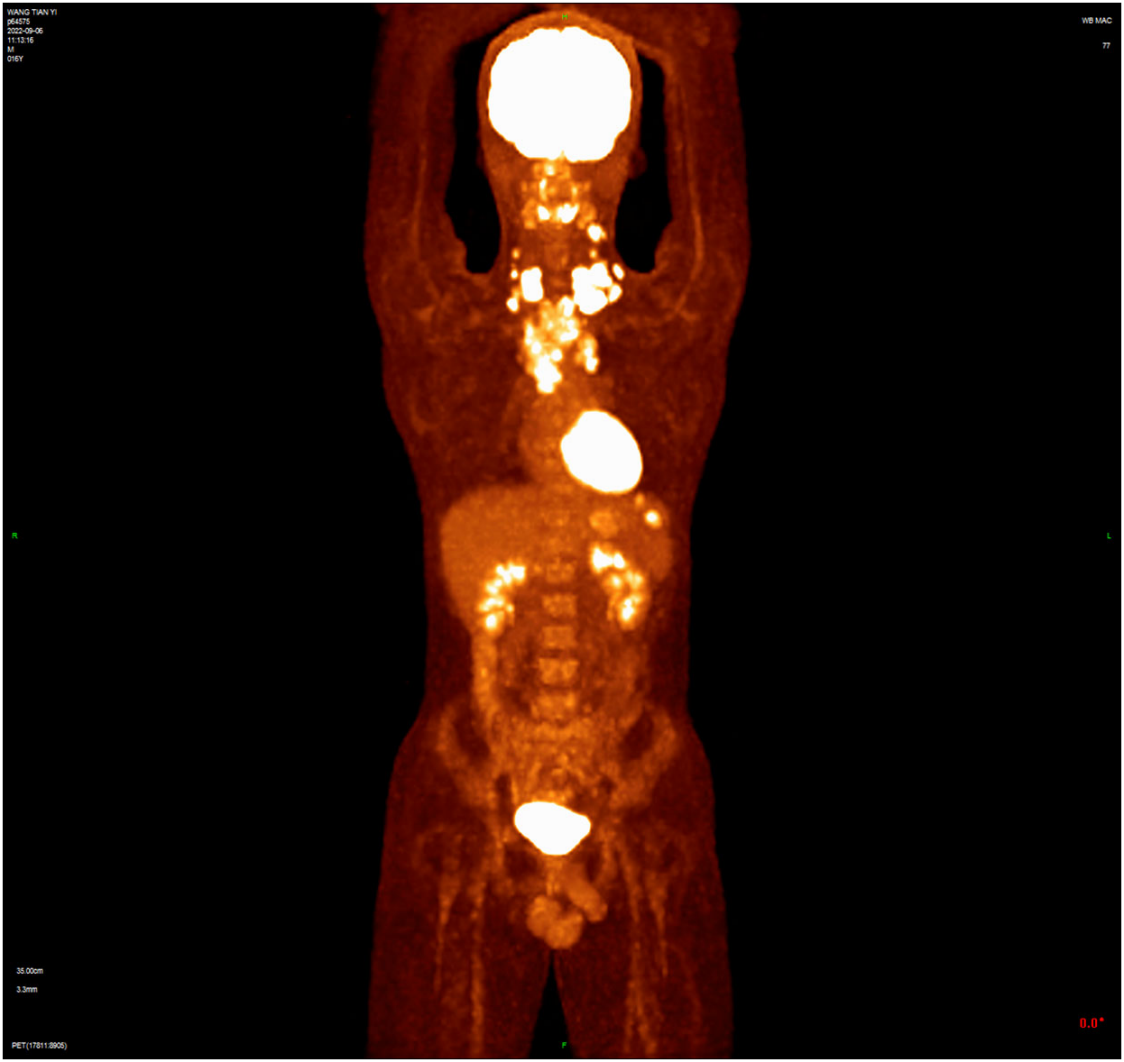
The baseline PET/CT shows that tumor had invaded the cervical and thoracic lymph nodes, spleen, and three cervical vertebrae.
